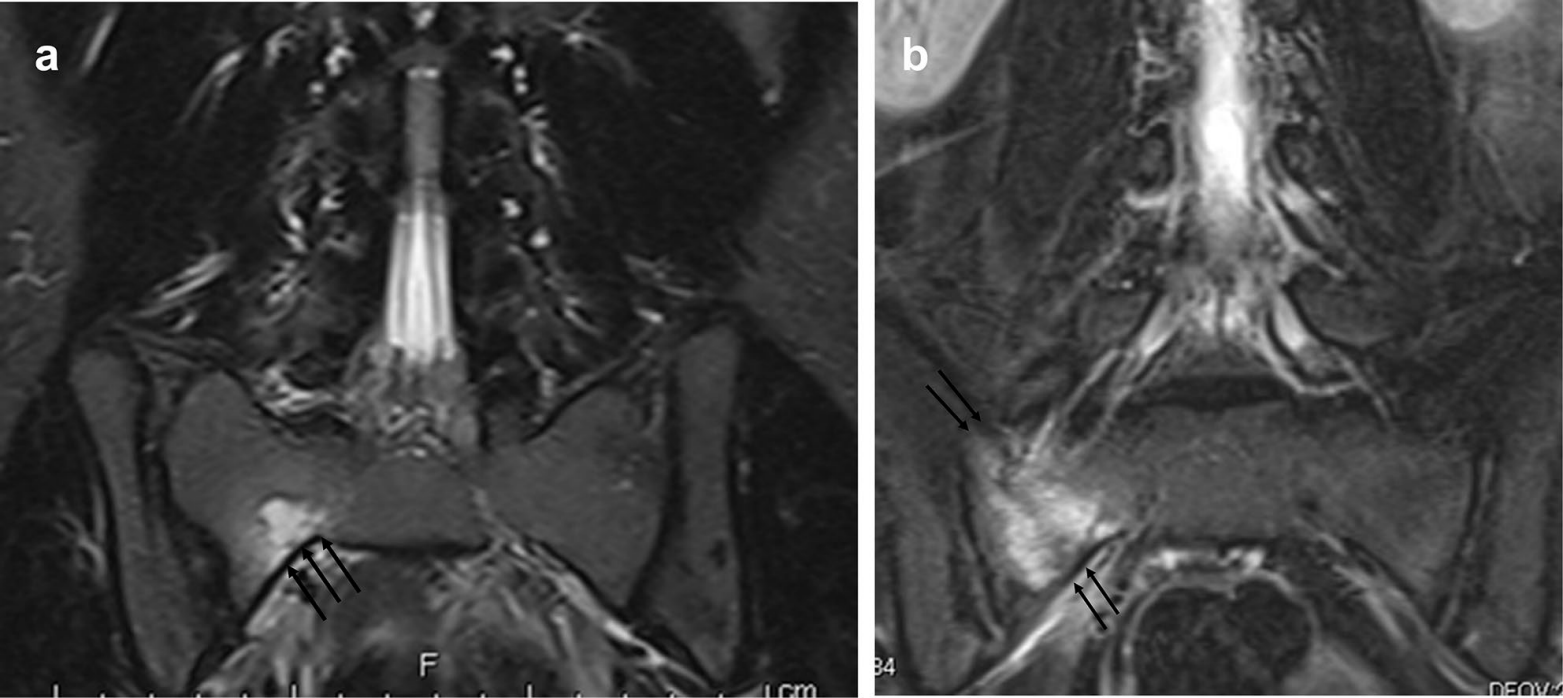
Characteristic of the area of bone marrow edema on MRI STIR. (a) This is a sample view of bone marrow edema that existed locally around the superior sacral foramen on the MRI STIR coronal view (arrows indicate bone marrow edema). (b) This is a sample view of bone marrow edema extending from the superior anterior sacral foramina to the superior lateral sacral alar parallel to the sacroiliac joint on the MRI STIR coronal view (arrows indicate bone marrow edema).
